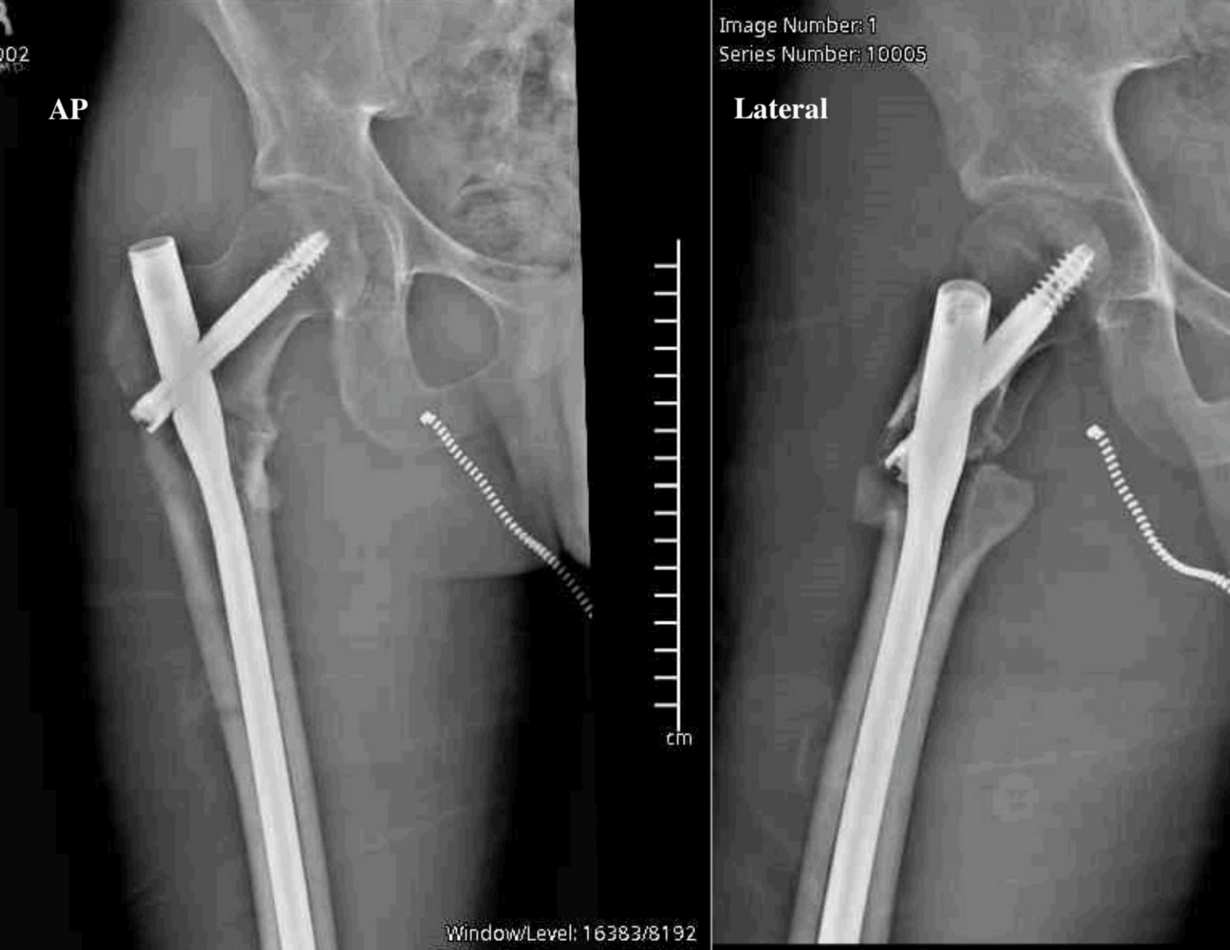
Anteroposterior (AP) and lateral radiographs of right hip eight weeks postoperatively showing concentrically reduced hip joint and acceptable fracture reduction after closed reduction of inferior hip dislocation and closed reduction and intramedullary nailing of associated intertrochanteric fracture
